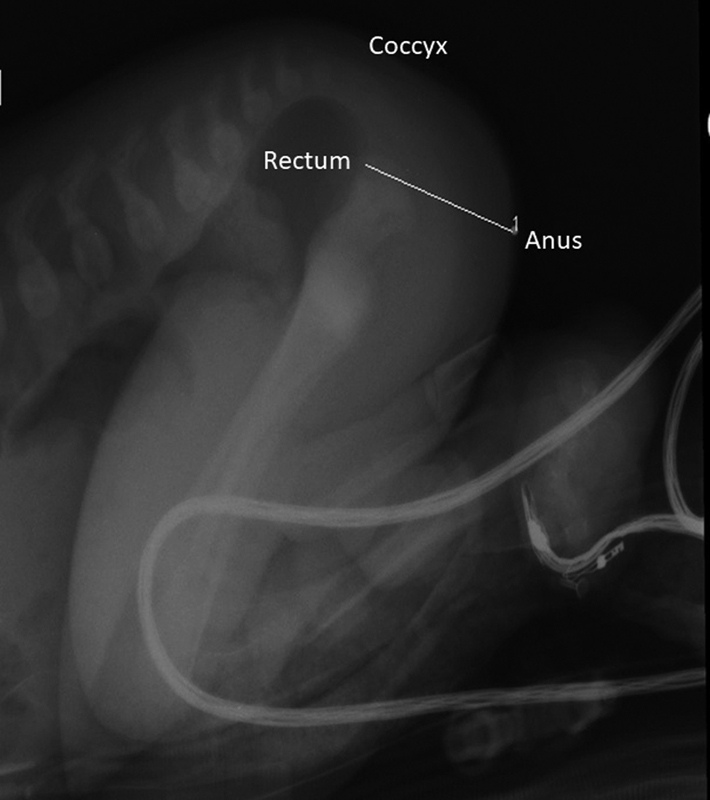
Cross-fire X-ray showing air column in rectum in relation to perineal skin and coccyx.
